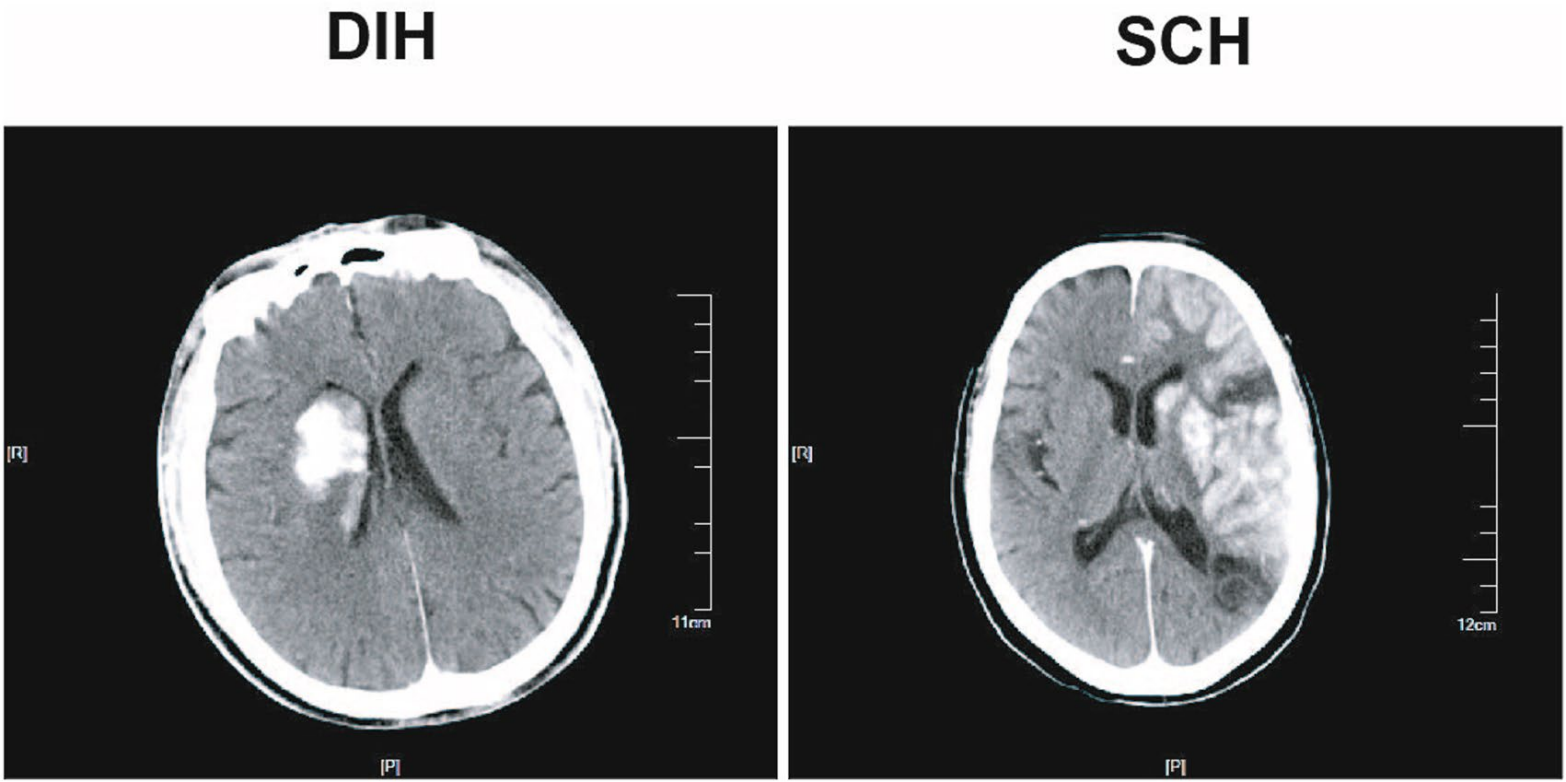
DIC and SCH on cranial NCCT images within 0.5 h after MT. DIH, deep intraparenchymal hyperdensities; SCH, subarachnoid/cortical hyperdensities; NCCT, non-contrast computed tomography; MT, mechanical thrombectomy.
